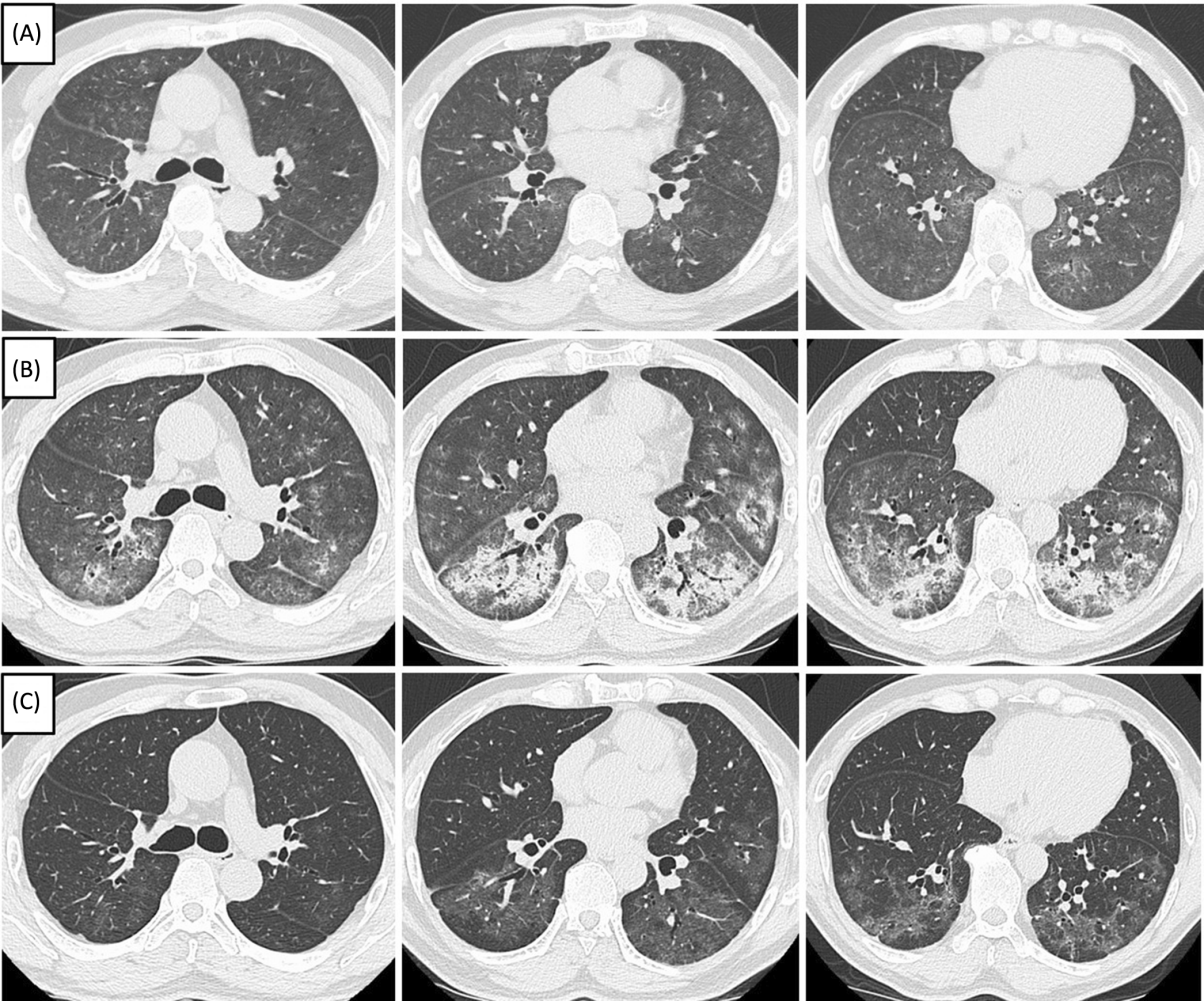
Images of chest CT at the first admission (A), at the readmission (B), and 2 weeks after starting the corticosteroid treatment (C).
